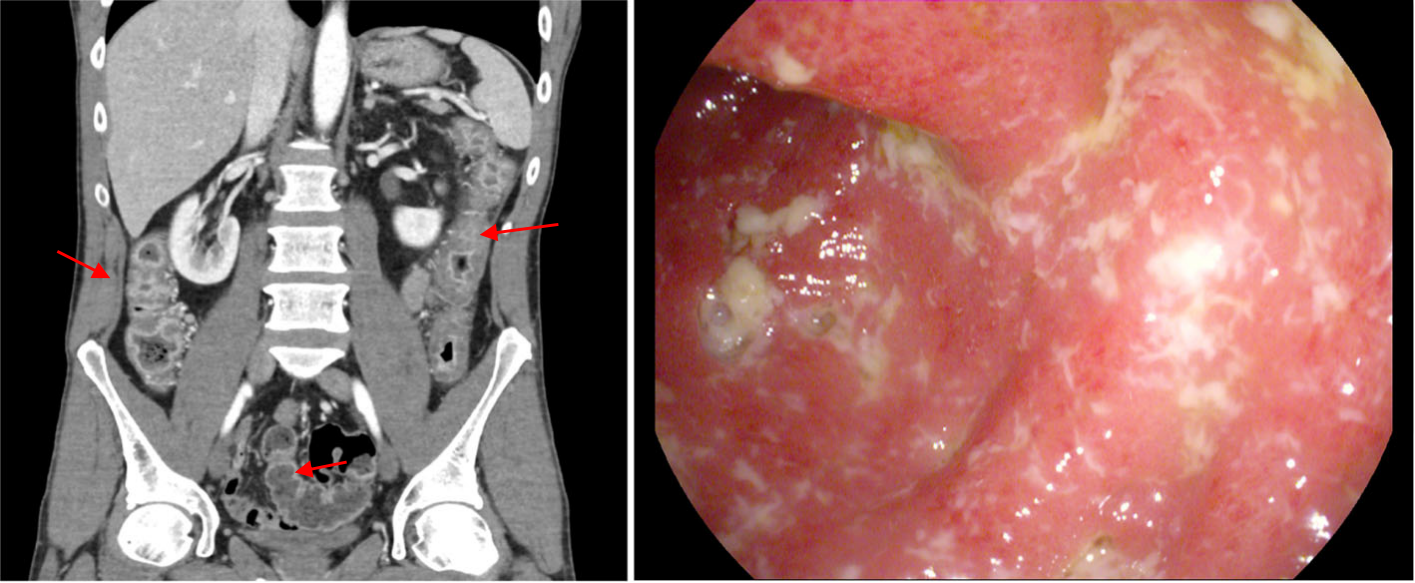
Abdominal CT showing mural thickening from ascending colon, to distal descending colon, with relative rectal and sigmoid sparing (Left). Arrows clockwise highlight descending colon, rectum and ascending colon. Representative image of ascending colon at index colonoscopy (Right)
